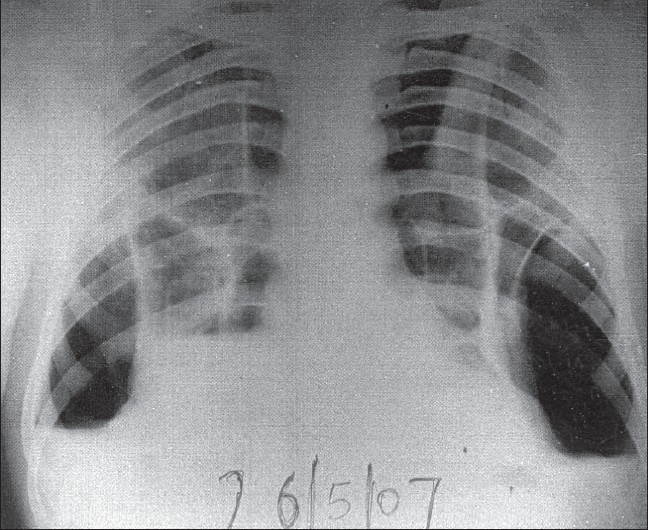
Repeat chest radiograph revealing bilateral air-fluid levels, suggestive of bilateral loculated pyopneumothorax
